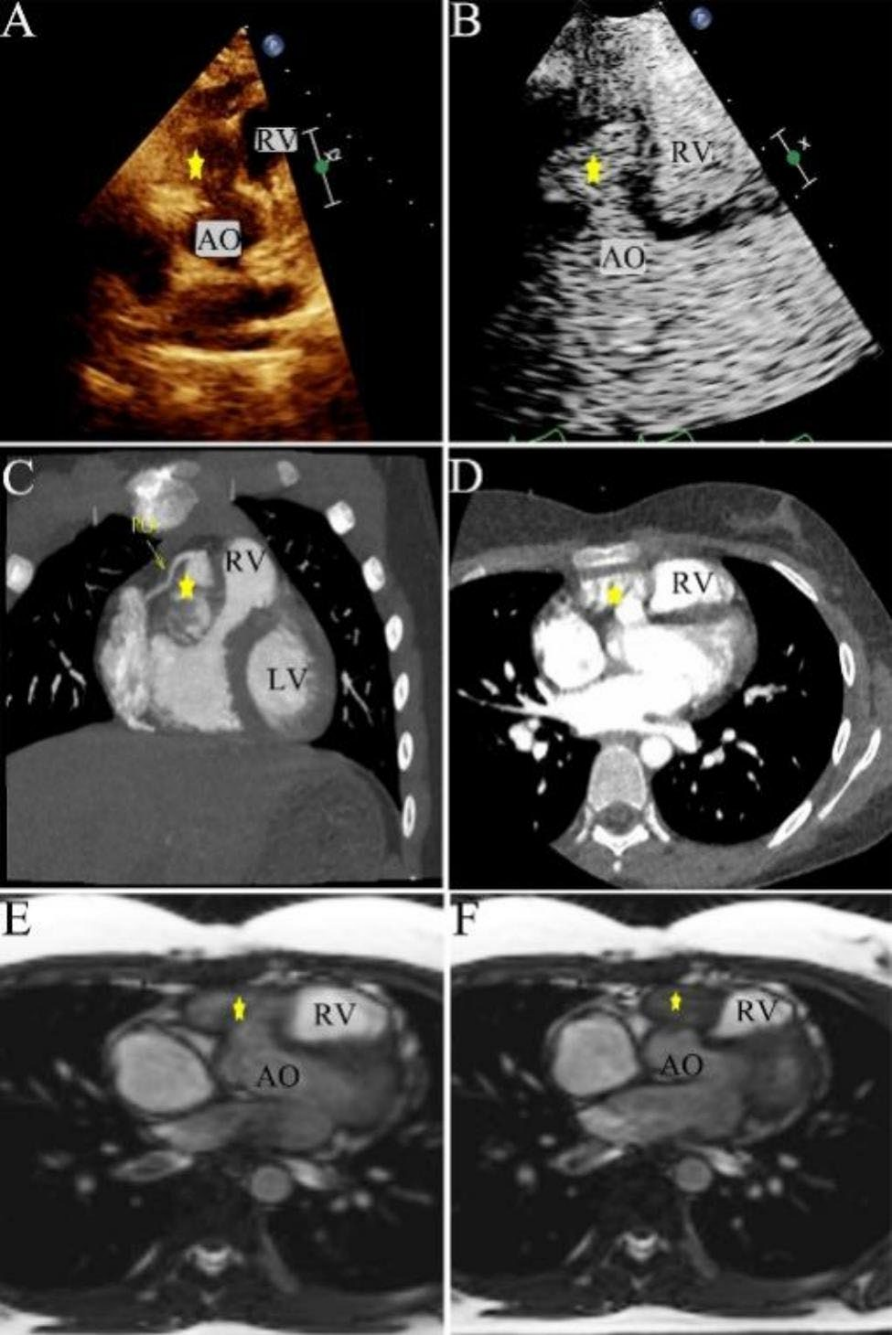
A. The echocardiography showed a bulge size of 21*13mm; B. The contrast echocardiography with sono vue showed a cystic structure opened under the aorta; C. The aneurysm had a close relationship to the right coronary. D. The aneurysm bulge to the right ventricle in enhanced-cardiac CT; E-F. Short-axis cine sequences of CMR showed a cystic shadow in front of the RV, E. at the end of diastole, F. at the end of systole; The cystic structure is designated by the yellow star. LV left ventricular; RV right ventricular; AO, aorta; RCA, right coronary artery
